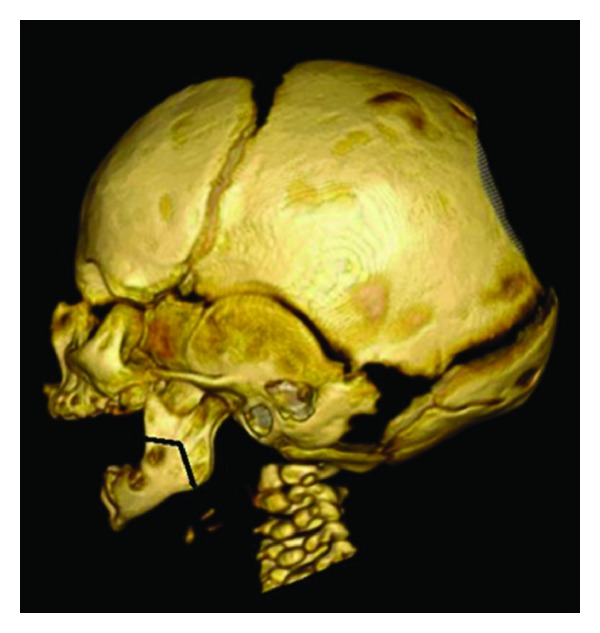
A lateral view of the three-dimensional reconstructed computed tomographic image of an infant with micrognathia-associated upper airway obstruction. Note the inverted L-shaped osteotomy design on the left mandible to avoid the tooth buds. The internal distractor device footplates are secured on the lower aspect of the osteotomy.
